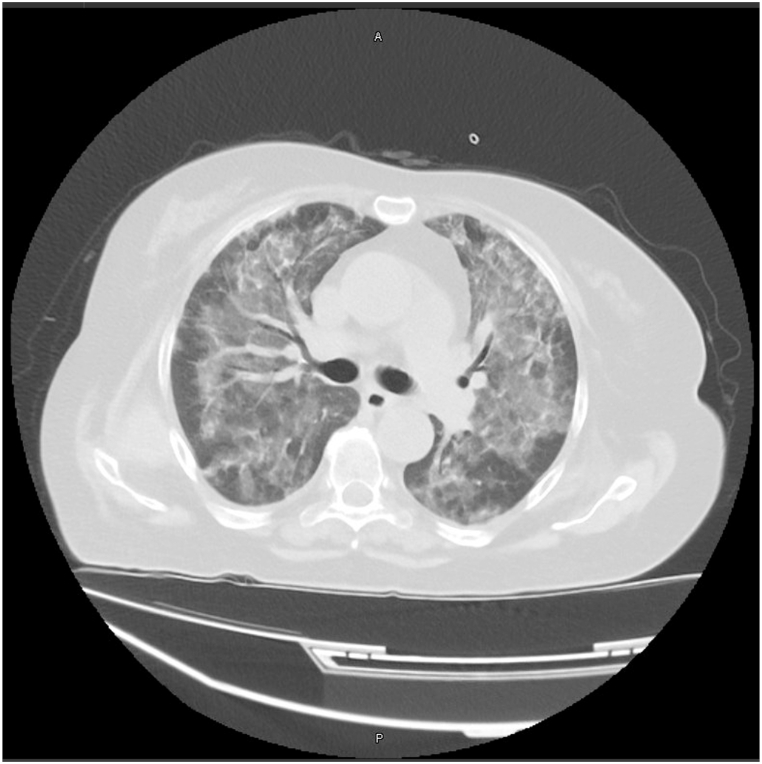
Axial CT scan taken before Mesalazine was discontinued, demonstrating diffuse consolidation of the lungs with ground-glass lesions.
